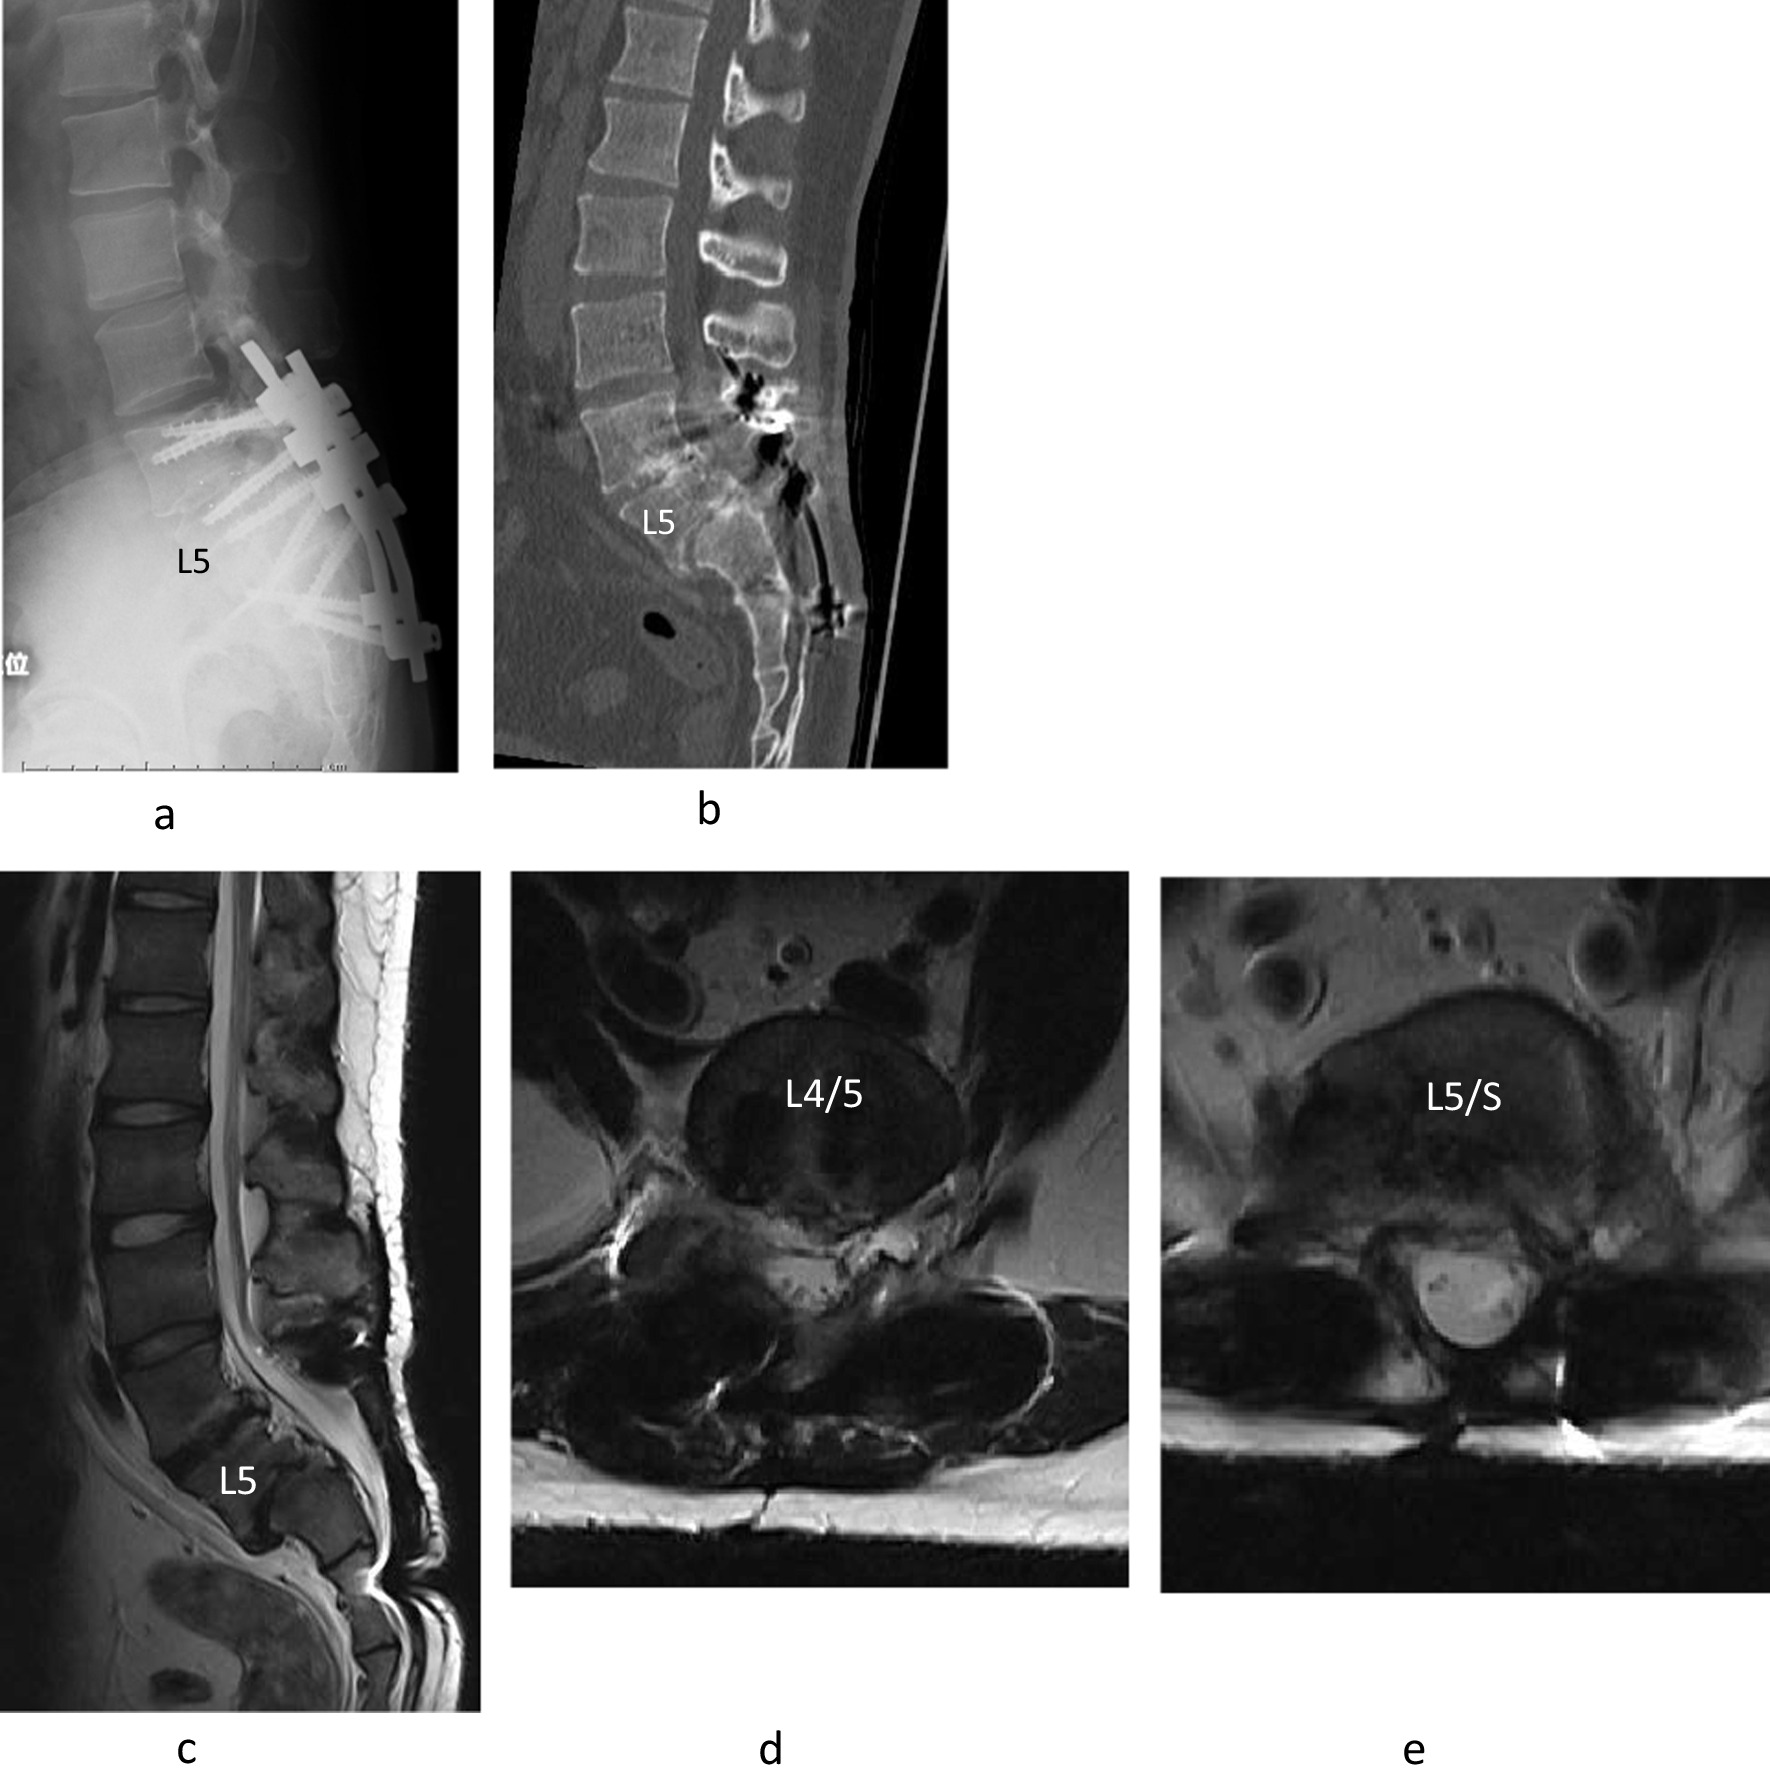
Posterior lumbar interbody fusion at L4/L5 and L5/S1 with reduction was performed on the younger brother with L4, L5, and S1 pedicle screws and S2 alar screws (a). Computed tomography indicated bony fusion between L5/S1 (b). MRI revealed that decompression was established (c–e) 5 years after surgery
